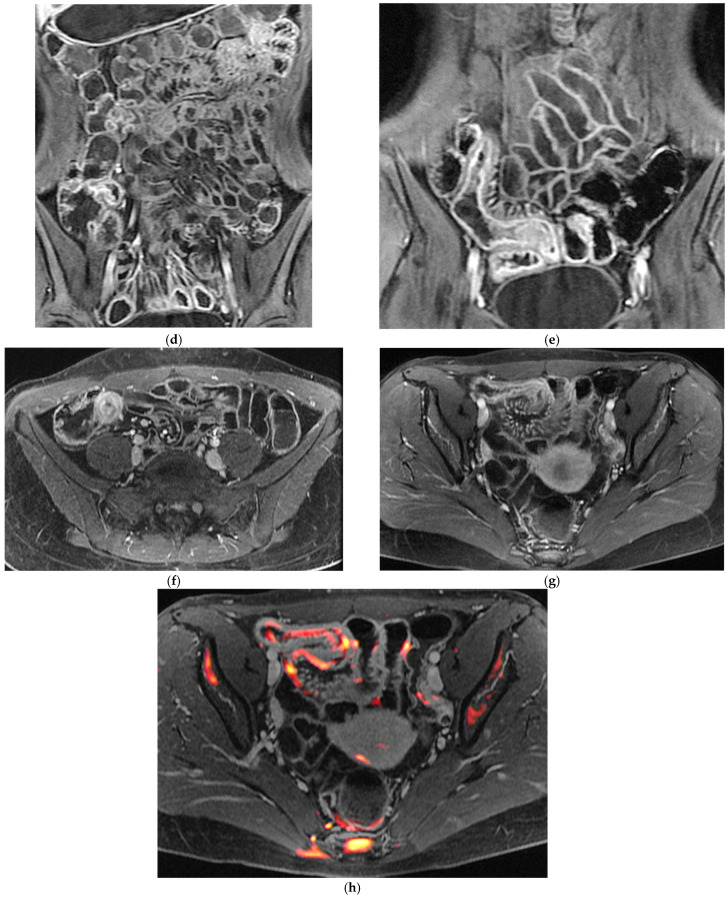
20-year-old female patient with typical Crohn’s disease of the terminal ileum, evaluated with a 3T Magnet, following oral ingestion of 1500 cc of PEG solution. (a): Coronal T2w images obtained with breath-hold acquisitions show diffuse inflammatory thickening of the last ileal loop exceeding 15 cm in length. Note how the remaining loops of the small bowel wall appear normal in contrast with the affected ileal loop. (b,c): axial T2 w images showing pathological thickening of the last ileal loop. (d,e): coronal T1-weighted scan after gadolinium injection, showing marked enhancement of the ileocecal valve and terminal ileum wall. In particular, an increased mucosa enhancement is clearly visible in the terminal ileum, well distinguishable from the normal mucosa of the remaining ileal and jejunal loops. The terminal ileum is affected for at least 15–20 cm; local mesenteric hyperaemia is also observed. The entire small bowel is fully displayed (d), including jejunal and ileal loops, which appear normal with respect to the affected terminal ileum. (f,g): Axial Gd-enhanced T1-weighted images show marked concentric wall thickening and enhancement of the terminal ileum with associated mesenteric hyperaemia, due to severe inflammatory involvement. (h): Post-processing “fusion” image, in which the T2 image is merged with the post-contrast T1 image, highlighting the pathological ileal loop in 20-year-old female patient with Crohn’s disease.
